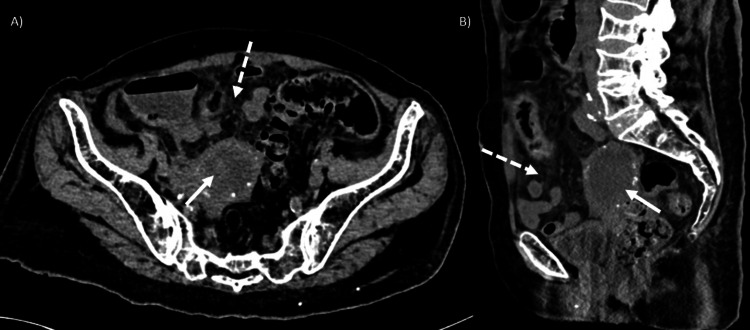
Computerized tomography in a simple phase of the abdomen. Axial (A) and sagittal (B) CT scans show a heterogeneous-appearing uterus with hypodense material in the endometrial cavity (thick arrow), associated with increased adjacent fat density (thick arrow).
